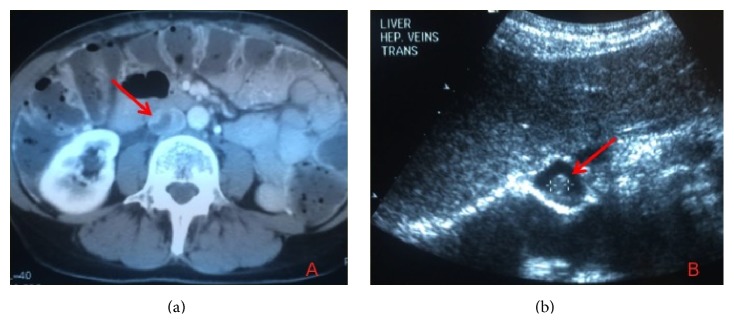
(a) Hypodense filling defect in the IVC on CT and (b) free-floating echodensity at the level of the hepatic veins, both consistent with intracaval thrombus.
